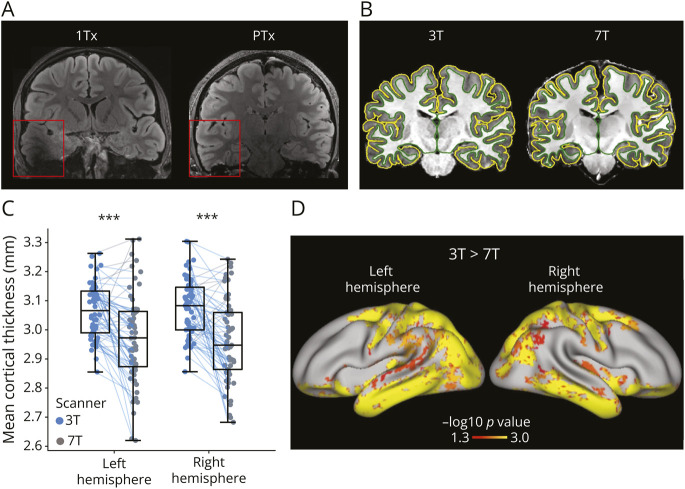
Imaging Characteristics of 3T and 7T MRI (A) Impact of parallel RF transmission on the FLAIR image of a healthy control. The image on the left, acquired with 1Tx, shows a visible signal drop in the right temporal lobe. No signal drop is present on the image on the right, acquired with PTx. (B) Example of a cortical delineation in a single participant at 3T and 7T. (C, D) Cortical thickness evaluation. Overall, the detected cortical thickness was thinner at 7T for both left (*t* = 5.01, *p* < 0.001) and right (*t* = 5.65, *p* < 0.001) hemispheres (C), with thinner boundaries in temporo-parietal/sensory regions (D). 1Tx = single RF transmission; FLAIR = fluid-attenuated inversion recovery; PTx = parallel RF transmission; RF = radiofrequency.
